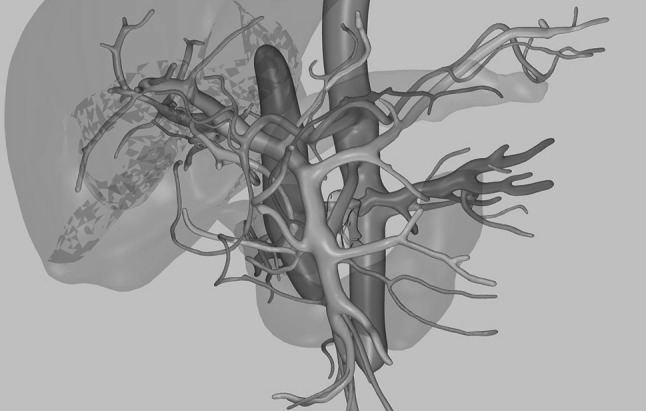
3D reconstruction of preoperative CT-scan for patient 2
